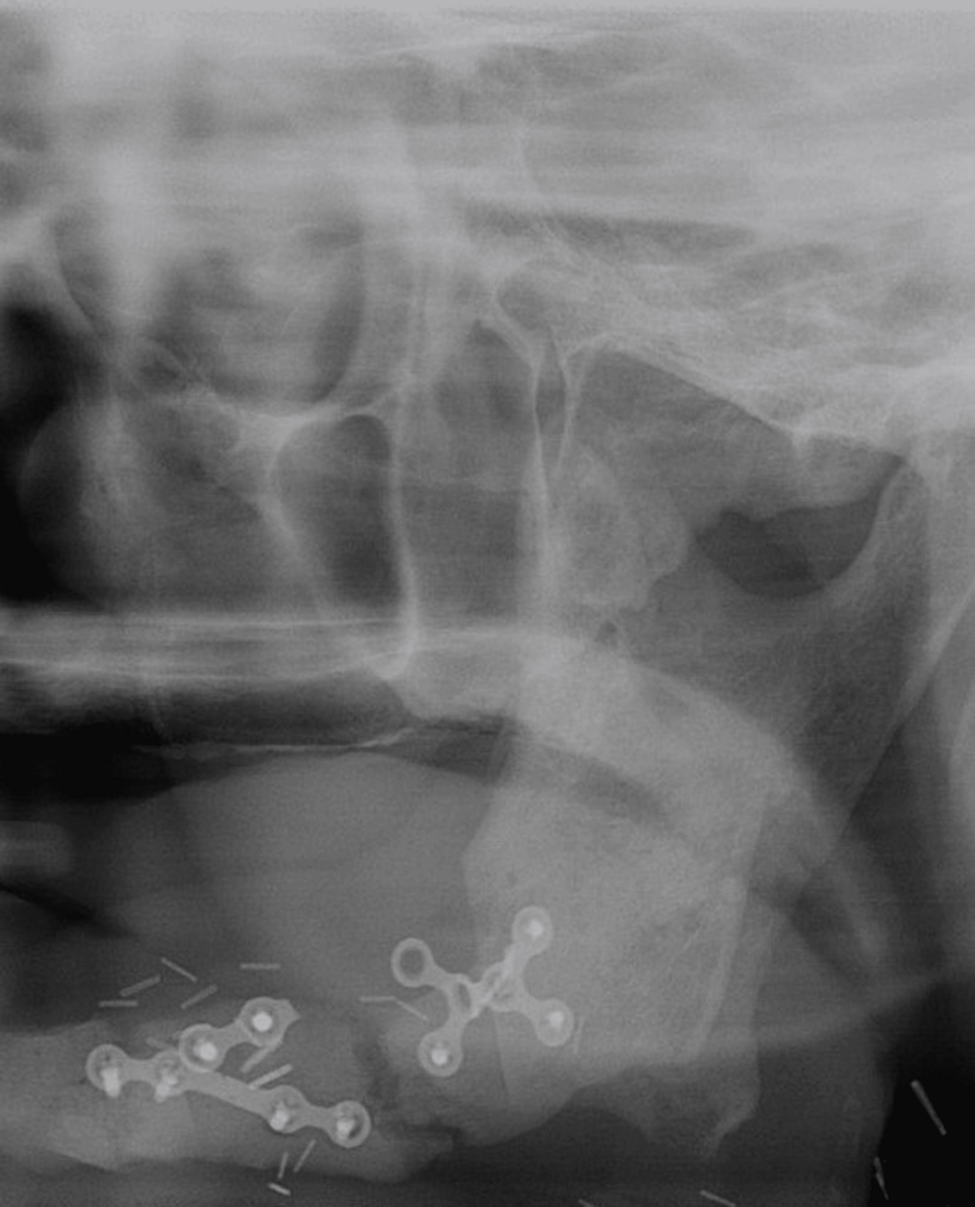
Panoramic radiography of a two-year postoperative follow-up Mandibular continuity was maintained for two years postoperatively. However, three screws and half of a plate were removed during the follow-up period due to loosening. New bone formation was observed, successfully connecting the bone segments.
